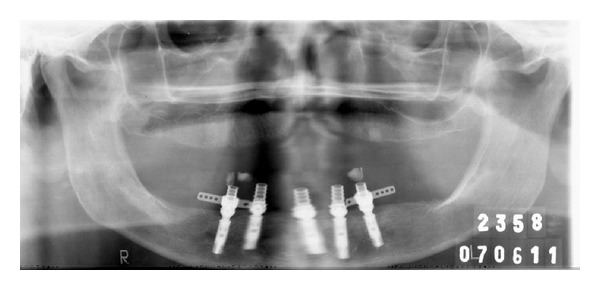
Panoramic radiography after 7 days of the prosthesis installation.
